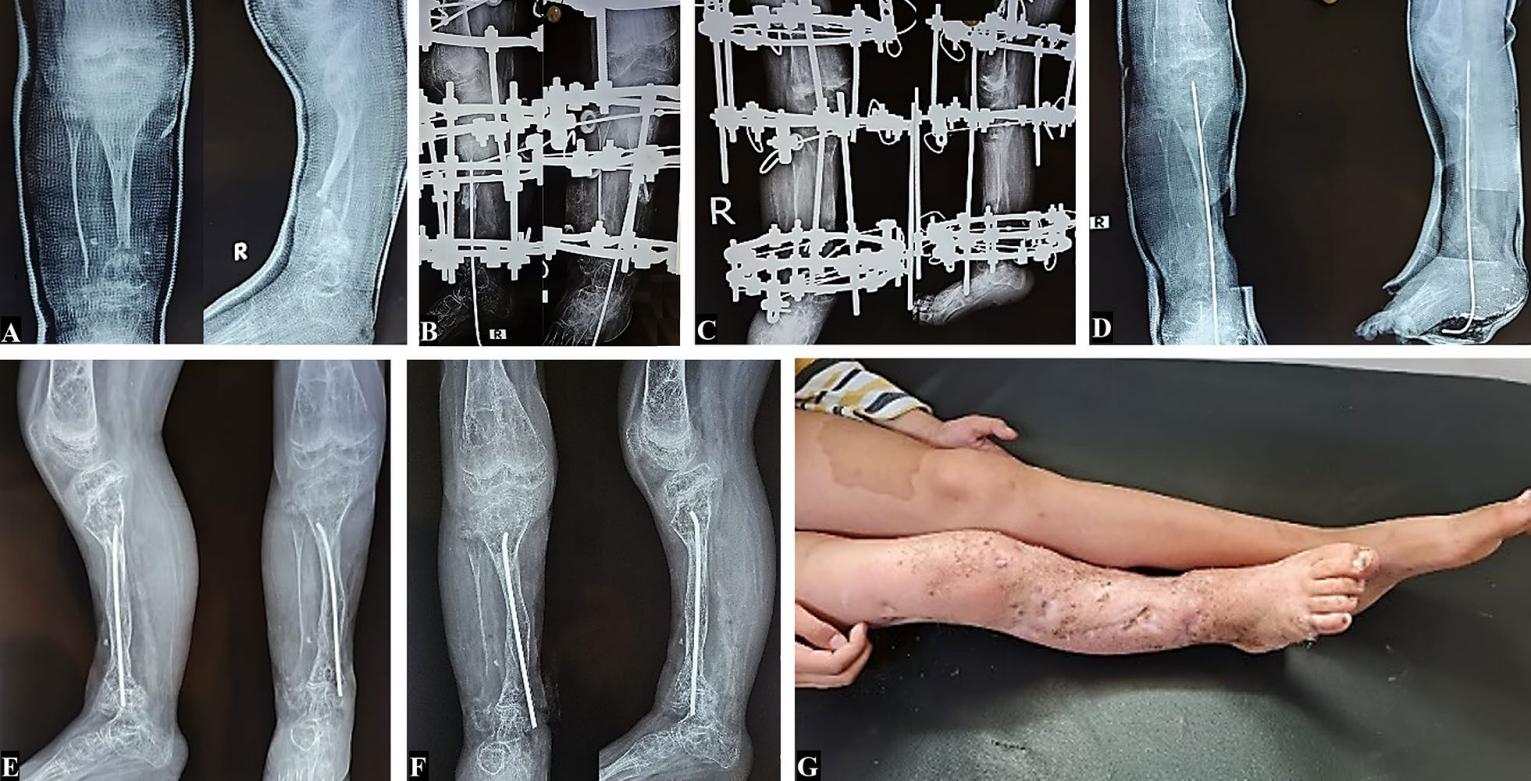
A Preoperative AP and lateral X-rays of a 7-year-old male who had undergone multiple previous surgeries for CPT since he was 1.5 years old, and had ended up with persistent CPT, posterior subluxation of the knee, genu recurvatum, and a very narrow, pencil-shaped tibia. B AP and lateral postoperative X-rays showing a trial correction of knee subluxation using a cross-knee Ilizarov frame, excision of the CPT, and compensation by proximal corticotomy. Note the relative widening of the end of the proximal tibia compared to the preoperative size. C Four months’ follow-up AP and lateral X-rays showing 6 cm distraction with a good regenerate. D AP and lateral X-rays taken immediately following fixator removal after full union with added transcalcaneal intramedullary K-wire for protection of the union. E 1.5 years’ follow-up AP and lateral X-rays showing complete union and consolidation of both the docking site and the regenerate, with widened tibia at the docking site and replacement of the K-wire by an IM titanium rod. F Two years' follow-up AP and lateral X-rays after changing the IM rod. G Clinical photograph taken at 2 years’ follow-up, showing 10 cm shortening of the affected limb
